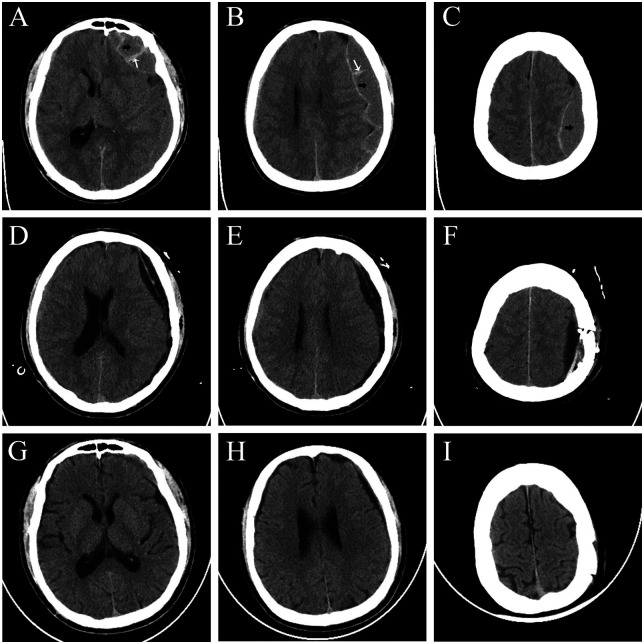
(A–C) sCSDH preoperative CT image, black arrow represents hematoma, white arrow represents fibrous septum inside hematoma. (D–F) CT imaging of the brain one week after endoscopic treatment. (G–I) CT imaging of the brain one month after endoscopic treatment.
